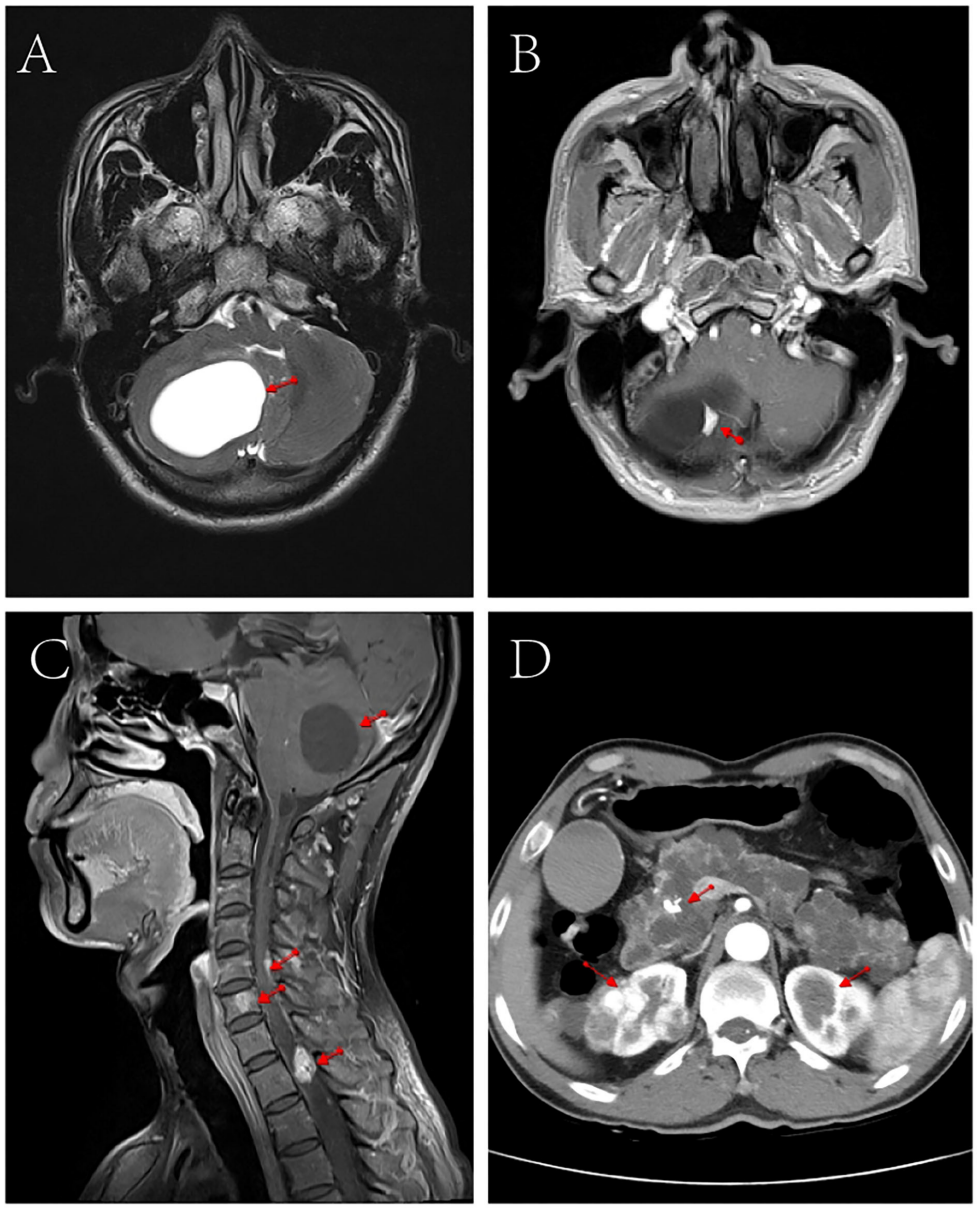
CT and MRI scan images of the patient. (A) Cranial MRI showed space- occupying lesions in the right cerebellar hemisphere. (B) Cranial enhanced MRI showed nodular enhancement in the right cerebellar hemisphere lesion (1.0 cm × 0.6 cm). (C) Contrast-enhanced MRI of the head and neck showed narrowing of the space in the fourth ventricle, multiple nodular and tortuous line-like enhancement in the spinal cord from C5 to T2, and round abnormal enhancement in the C6 vertebrae. (D) Abdominal enhanced CT showed multiple round soft tissue density lesions and multiple cystic low-density lesions in both kidneys, multiple low-density lesions of different sizes in the pancreas, and calcifications in the head of the pancreas.
